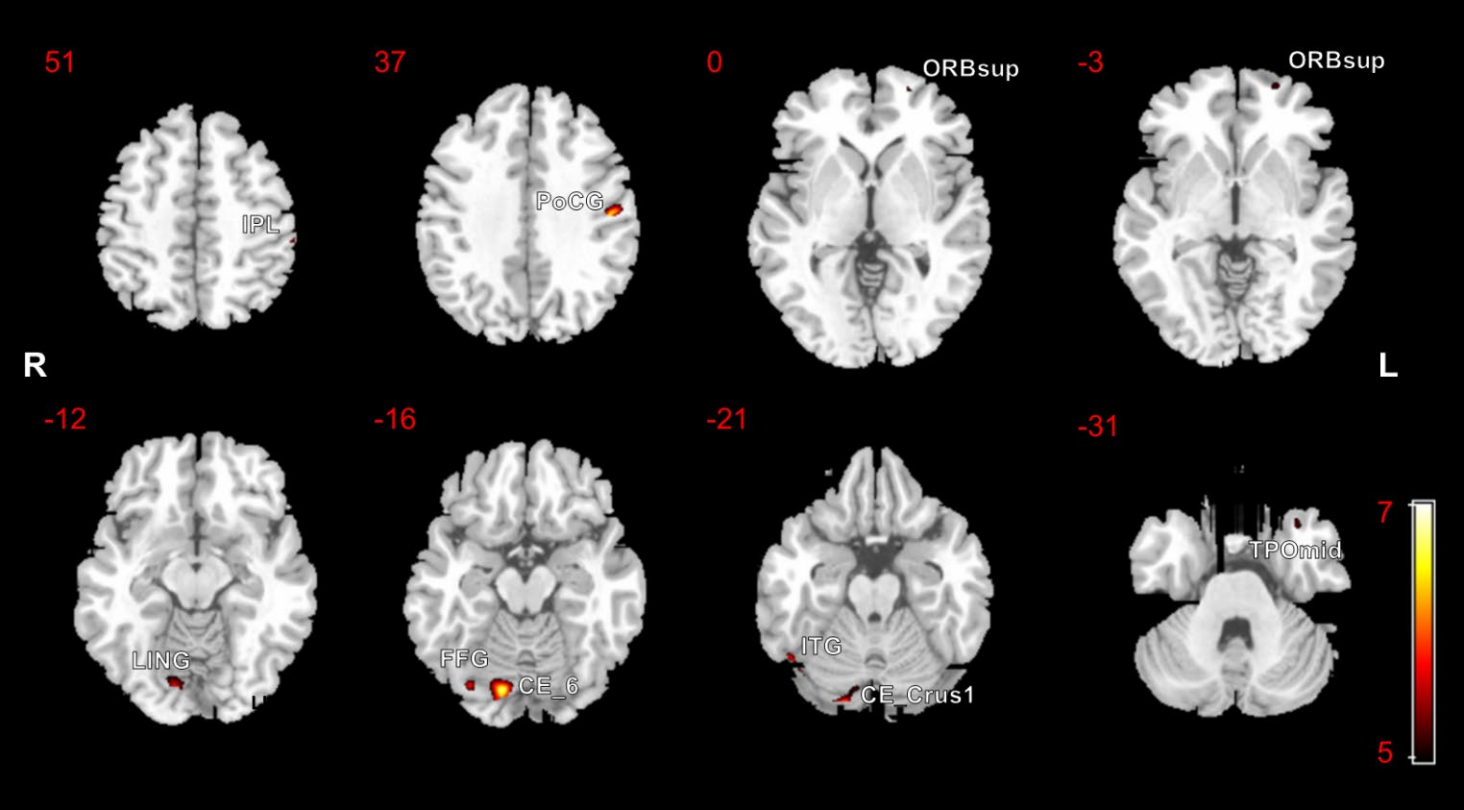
Statistical parametric mapping regions superimposed on a high-resolution T1-weighted scan show decreased grey matter volumes in patients with end-stage kidney disease and non-cognitive impairment, compared to the healthy controls (*p* < 0.05, family-wise error-corrected) R, right; L, left; IPL, inferior parietal but supramarginal and angular gyri; PoCG, postcentral gyrus, ORBsup, orbital part of superior frontal gyrus; FFG, fusiform gyrus; ITG, inferior temporal gyrus; LING, lingual gyrus; CE_6, cerebellum_6; CE_Crus1, cerebellum_crus1; TPOmid, temporal pole: middle temporal gyrus
